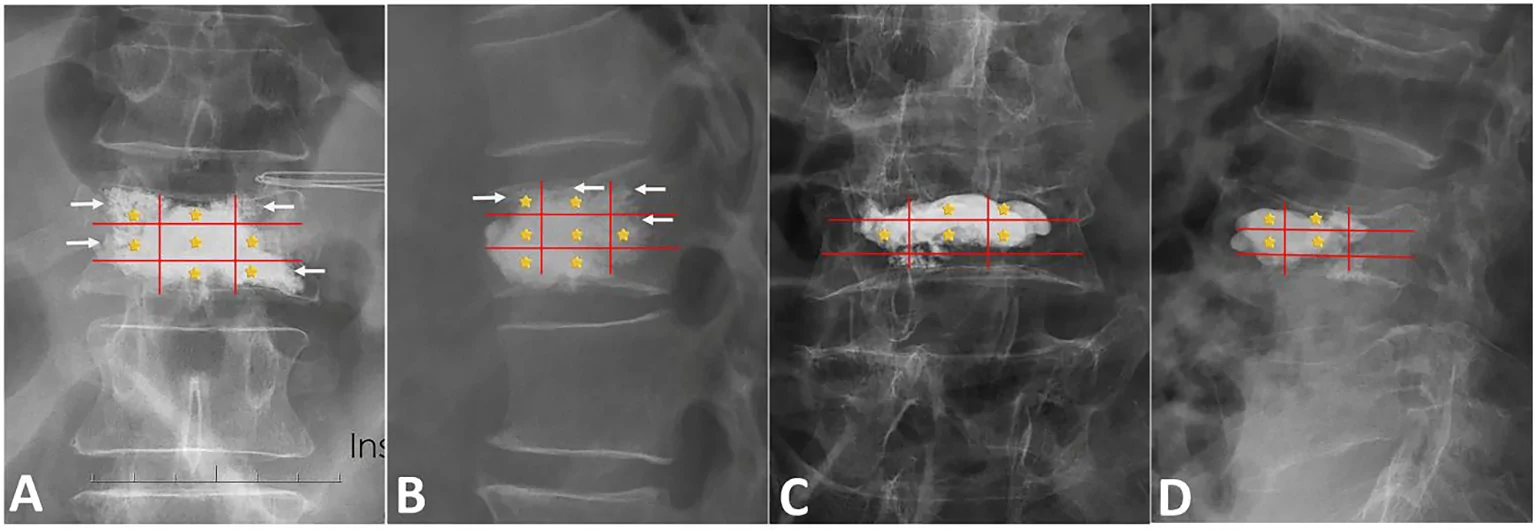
Spongy cement distribution method. The vertebral body was divided into 9 equal regions on post-operative anteroposterior and lateral X-rays. A region with > 50% cement filling was defined as an “effective” square (★). Dispersion into ≥ 6 effective squares was considered good. (A, B) Spongy pattern: bone cement interacted with cancellous bone (white arrow) and filled ≥ 6 effective squares. (C, D) Non-spongy pattern: bone cement did not interact with cancellous bone and filled < 6 effective squares.
